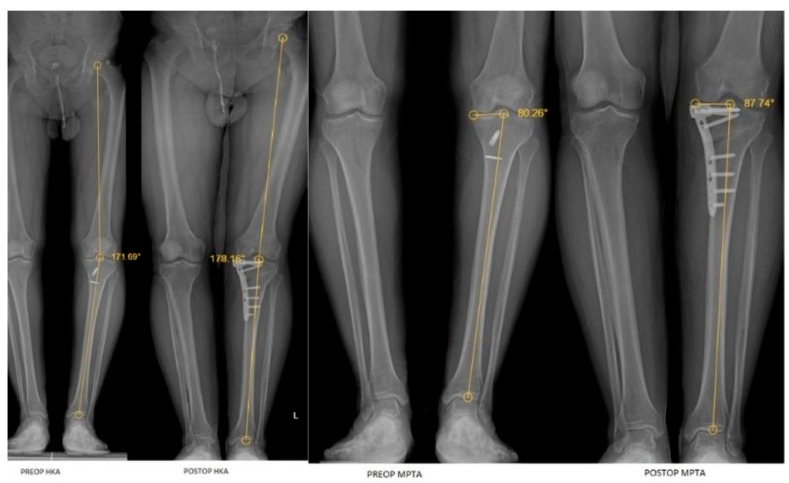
Preoperative and postoperative full-length lower-extremity radiographs showing knee and lower limb alignment measurements, including hip–knee–ankle angle (HKA) and medial proximal tibial angle (MPTA).
